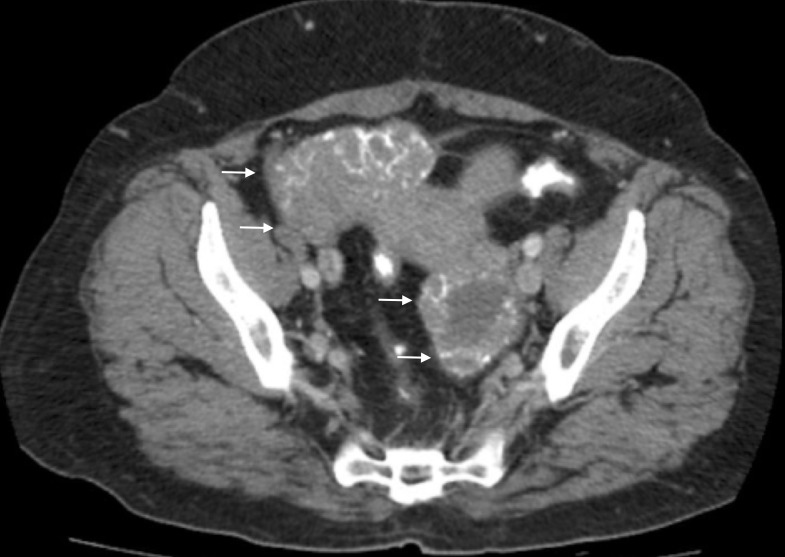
The additional image from the computed tomography (CT) of the abdomen and pelvis, performed in 2009, of the ovarian masses.
